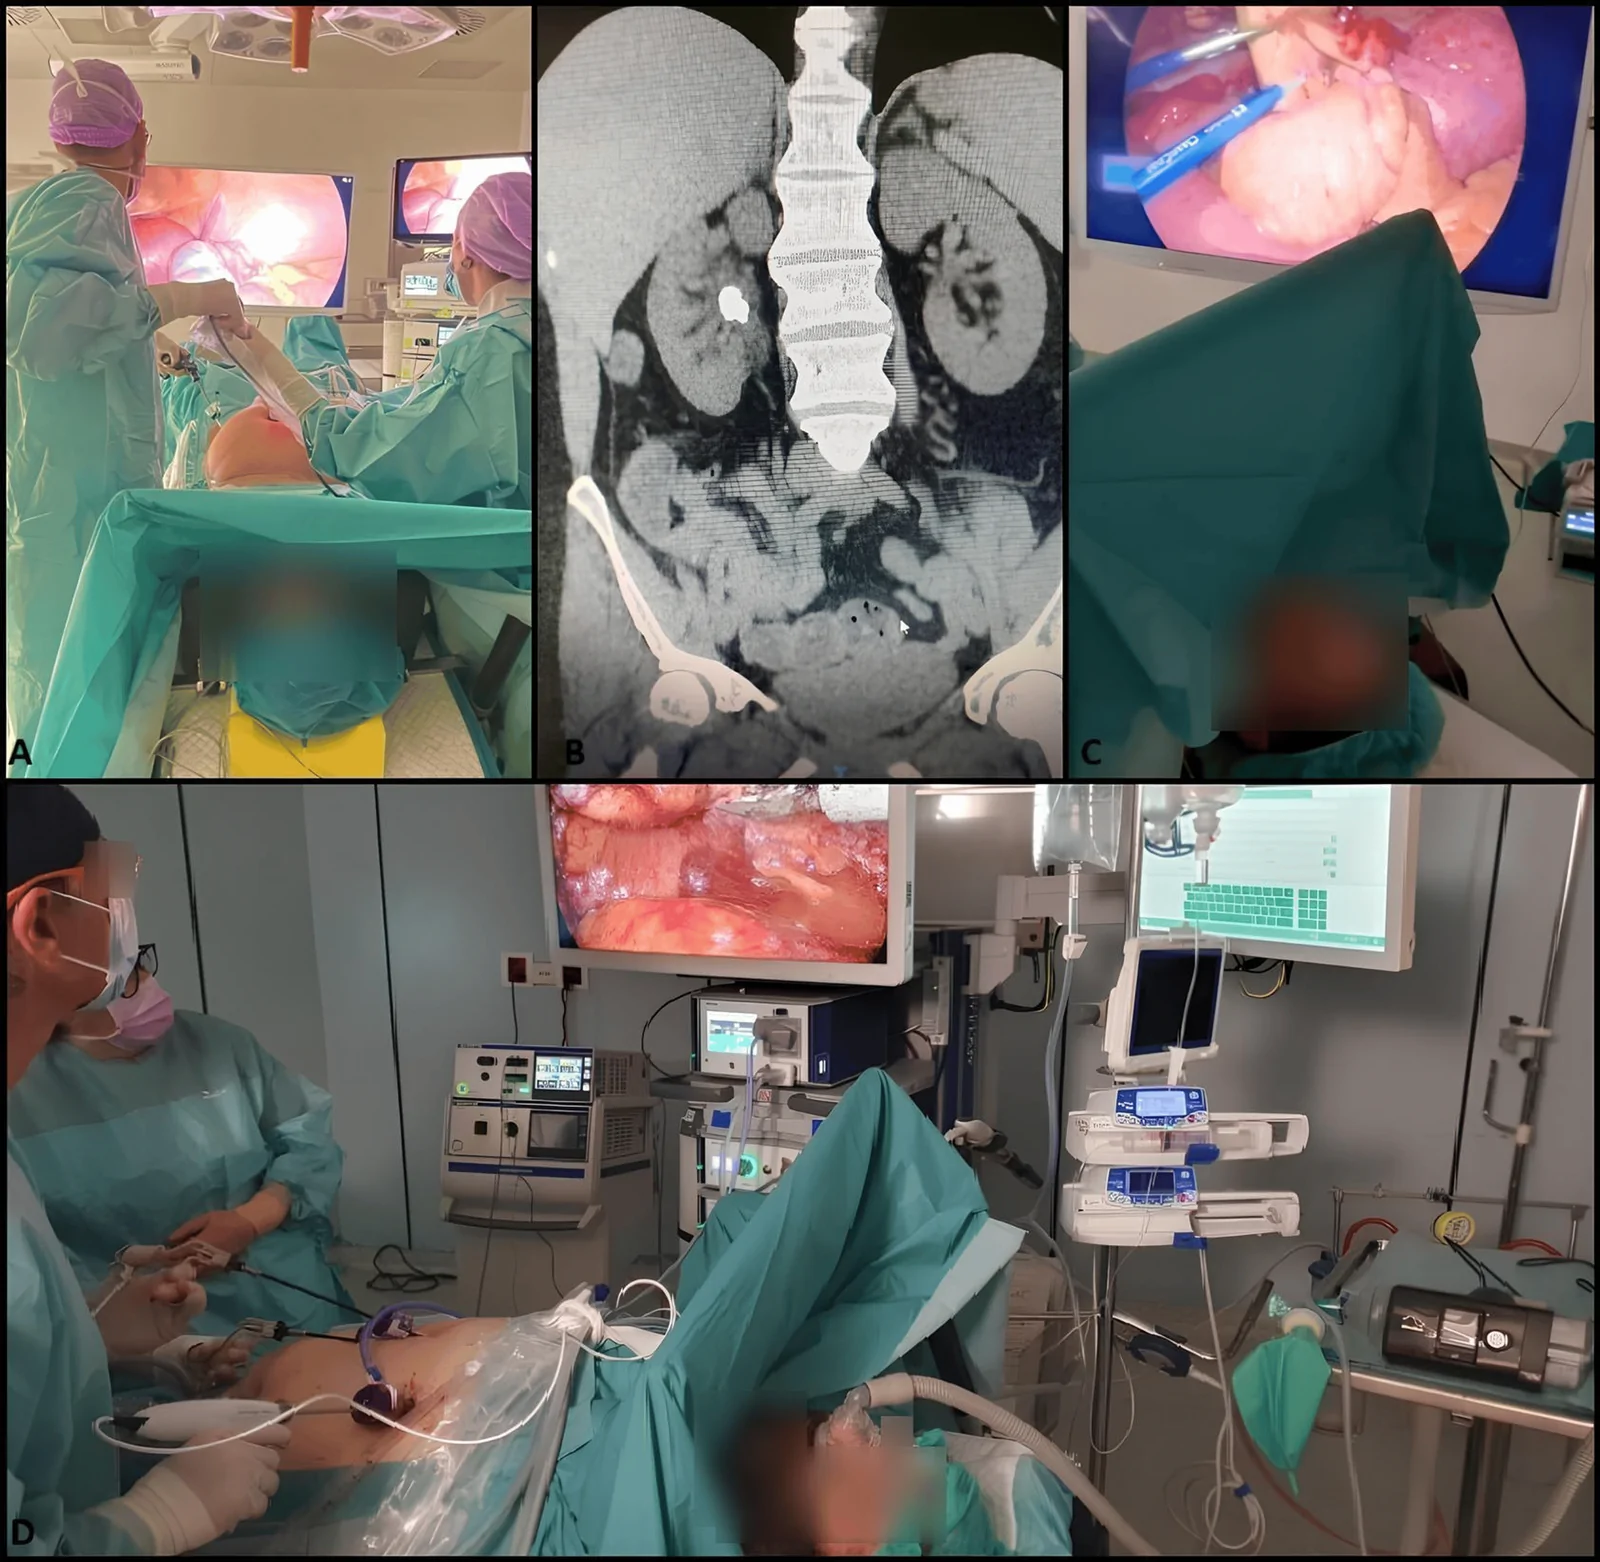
Representative image collage of the four cases managed with thoracic segmental spinal anesthesia (A) Case 1: Emergency videolaparoscopic adnexal detorsion in a first-trimester pregnant patient, performed under TSSA with preserved spontaneous breathing. (B) Case 2: Preoperative computed tomography image of right pelvic renal stones in a patient with arthrogryposis multiplex congenita and restrictive ventilatory impairment undergoing RIRS. (C) Case 3: Emergency laparoscopic appendectomy for acute gangrenous appendicitis in a patient with severe COPD, performed under TSSA while maintaining spontaneous breathing. (D) Case 4: Laparoscopic cholecystectomy in a morbidly obese patient with obstructive sleep apnea, performed under TSSA combined with CPAP support. TSSA: thoracic segmental spinal anesthesia; RIRS: retrograde intrarenal surgery; COPD: chronic obstructive pulmonary disease; CPAP: continuous positive airway pressure
